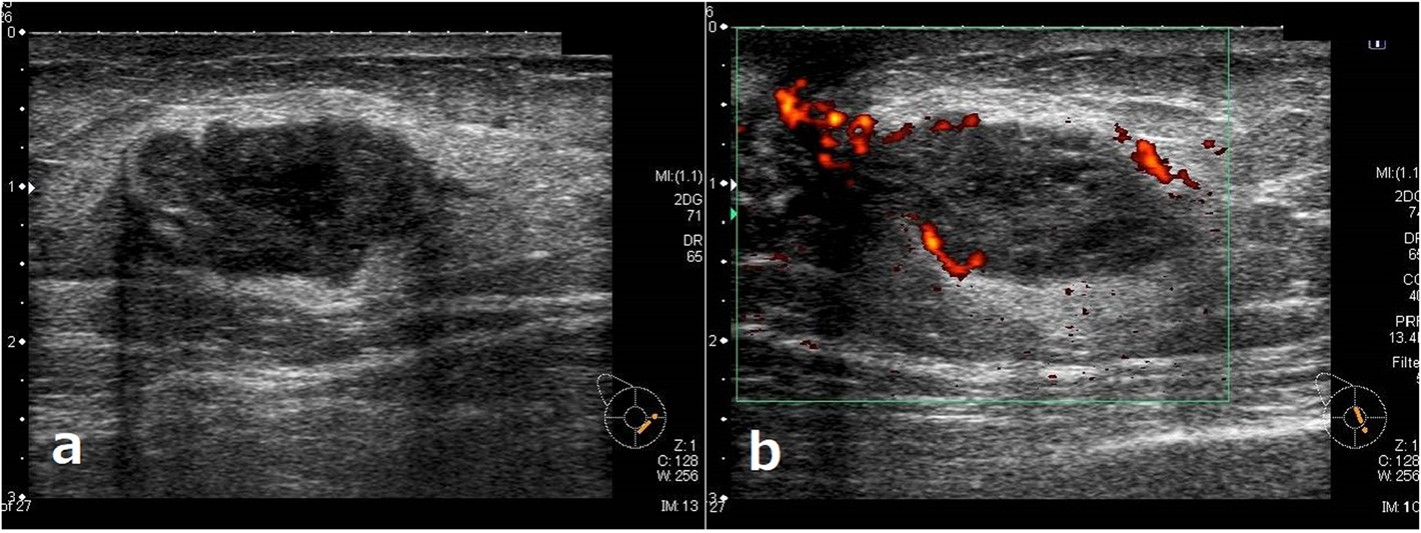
Breast ultrasonography. Legend: Breast ultrasonography showed an irregular, rough, internally inhomogeneous hypoechoic mass measuring 32 × 35 mm in the center of the right breast right beneath the nipple (a, b)
